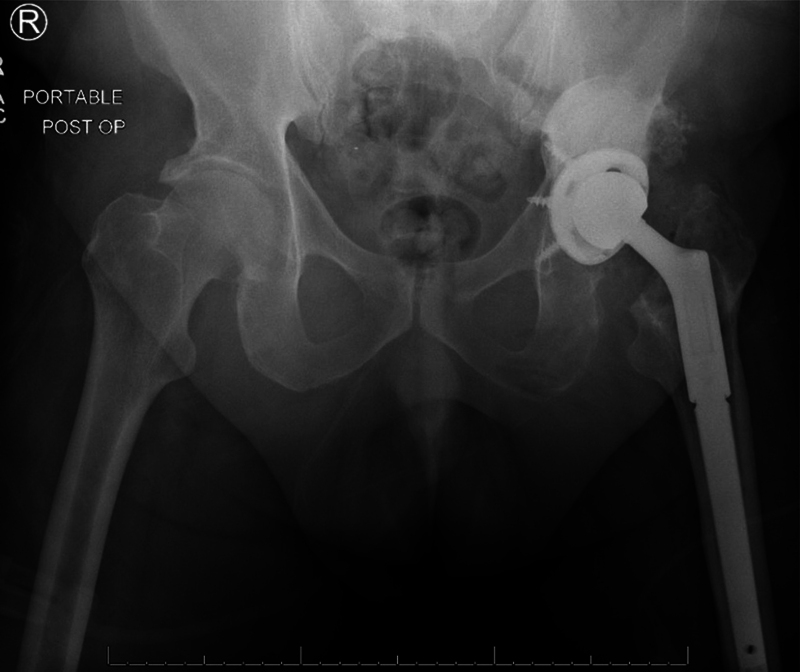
Anteroposterior radiograph of the pelvis showing a total hip arthroplasty as stage-2 revision.
